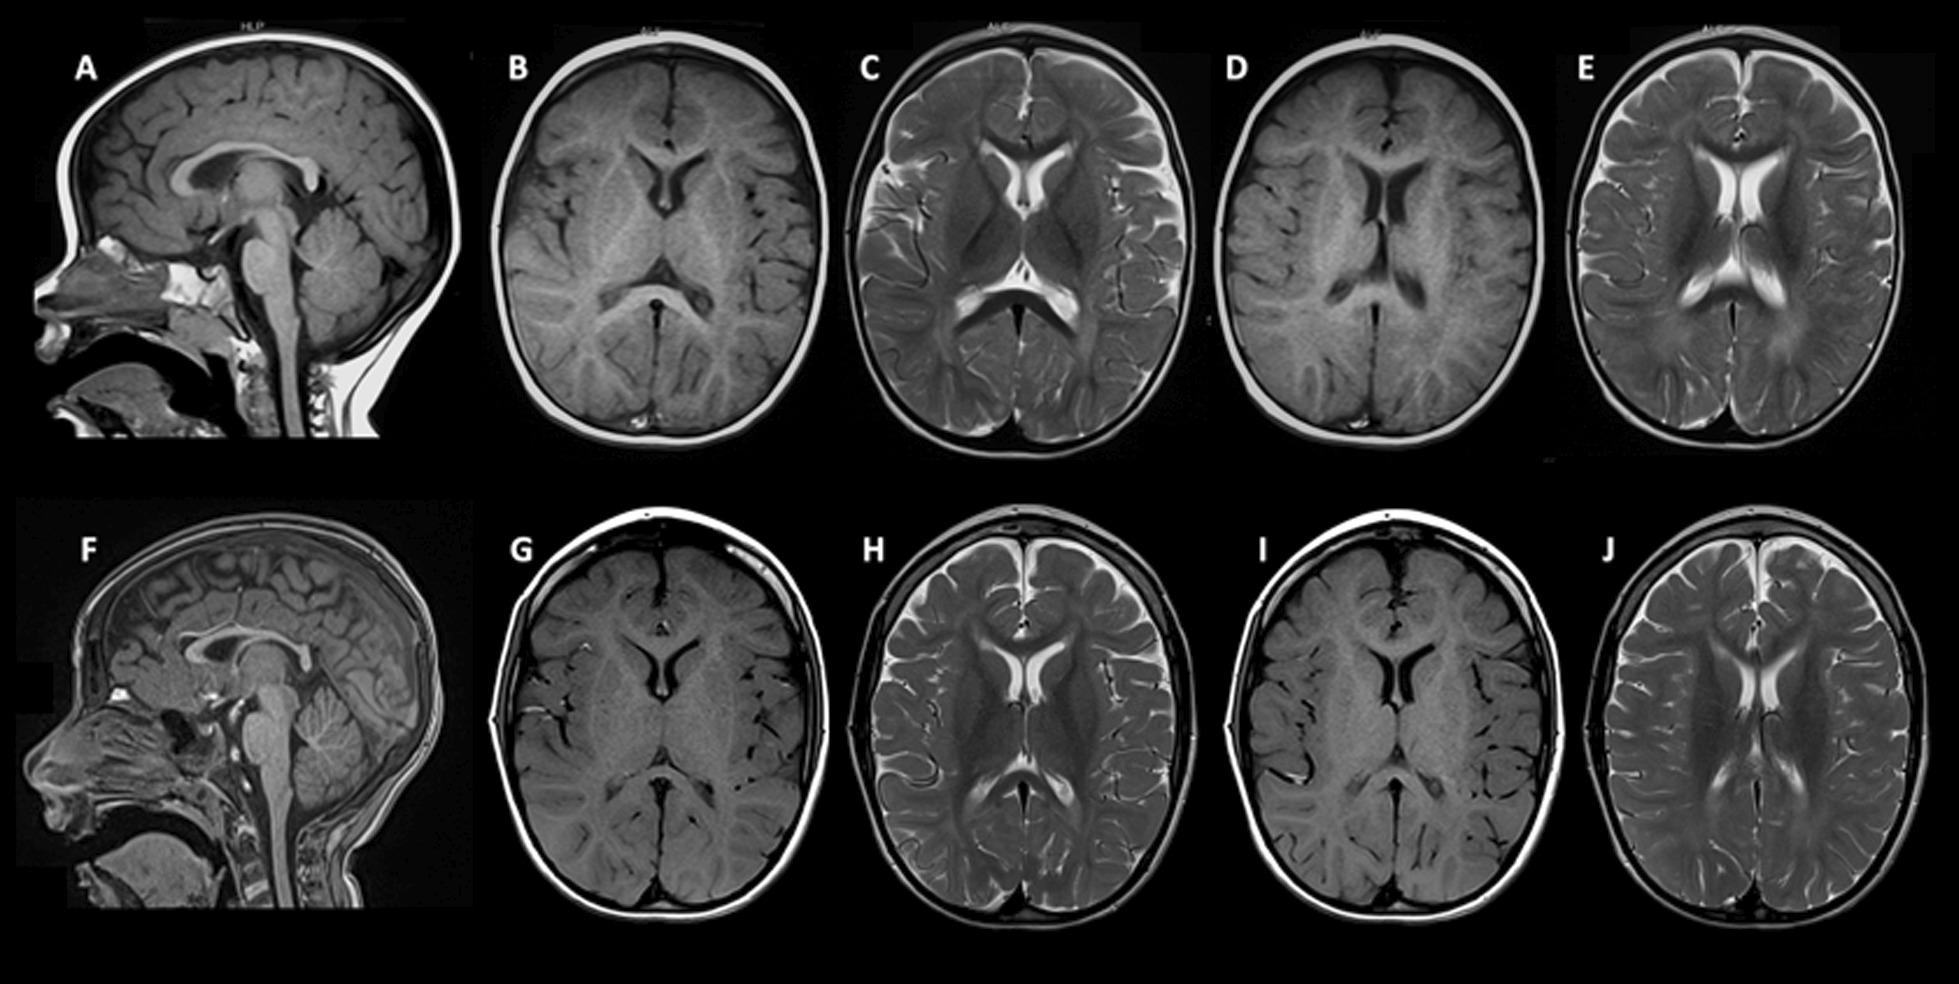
MRI at 14 months of age (A–E) and at 7 years of age (F–J). Thin corpus callosum was observed on sagital T1 sequence (A, F). Slight bilateral hypomyelination with hypointense T1 signal (B, D, G, I) and hyperintense T2 signal (C, E, H, J) in frontal and occipito-parietal periventricular and deep white matter, was observed
